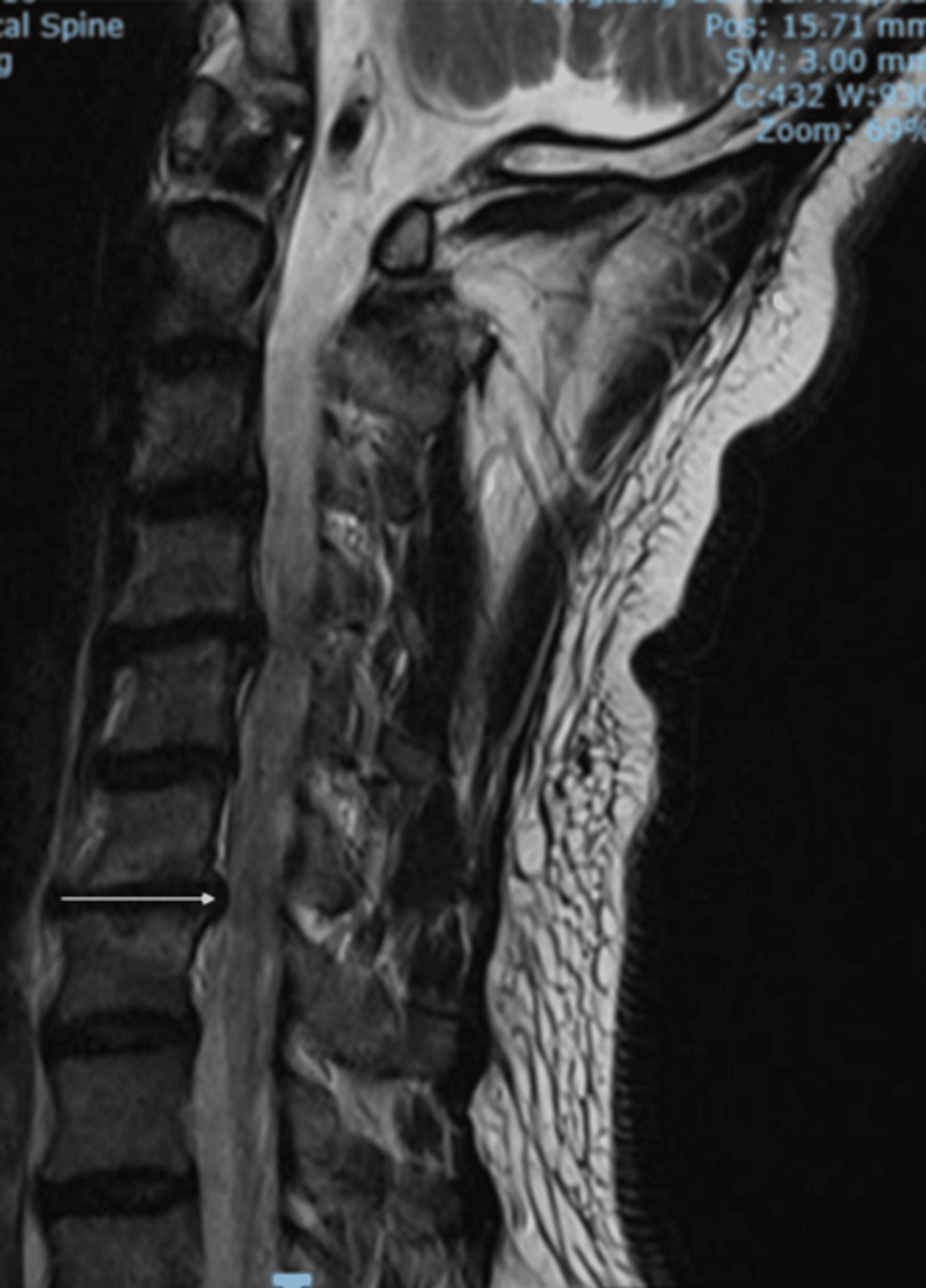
Preoperative T2-weighted MRI of the cervical spine (sagittal view) No spinal cord compression at C6/C7 (arrow) MRI: magnetic resonance imaging
